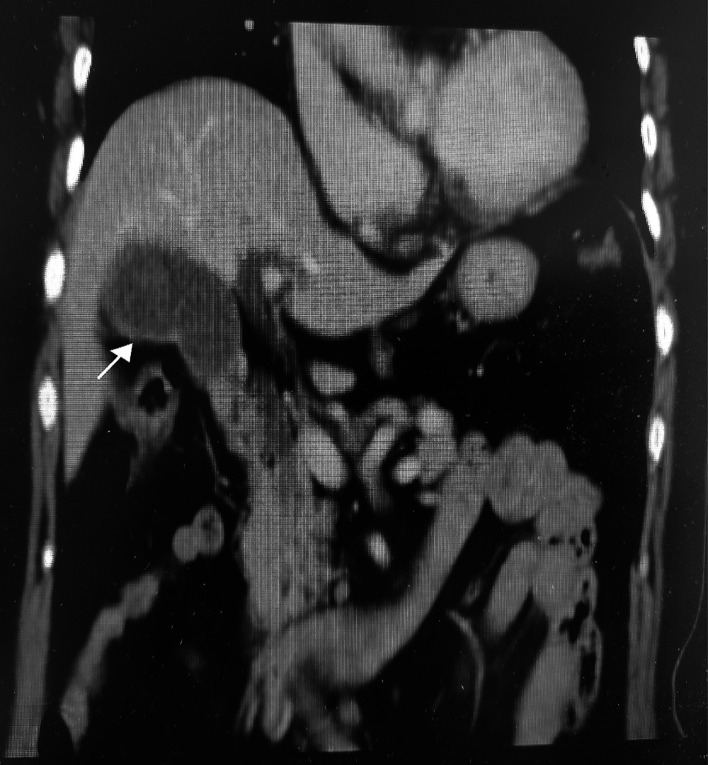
Representative coronal plane enhanced computed tomography (CT) image from a 66-year-old female patient with persistent pain for the previous 10 h, showing a typical-looking inflamed gallbladder (arrow) with marked distention and wall thickening.
